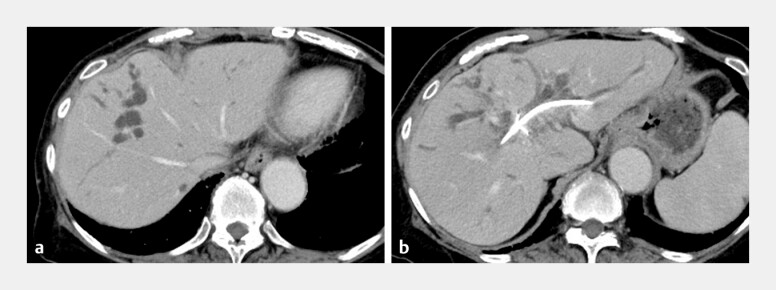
Follow-up computed tomography after EUS-guided hepaticogastrostomy.
